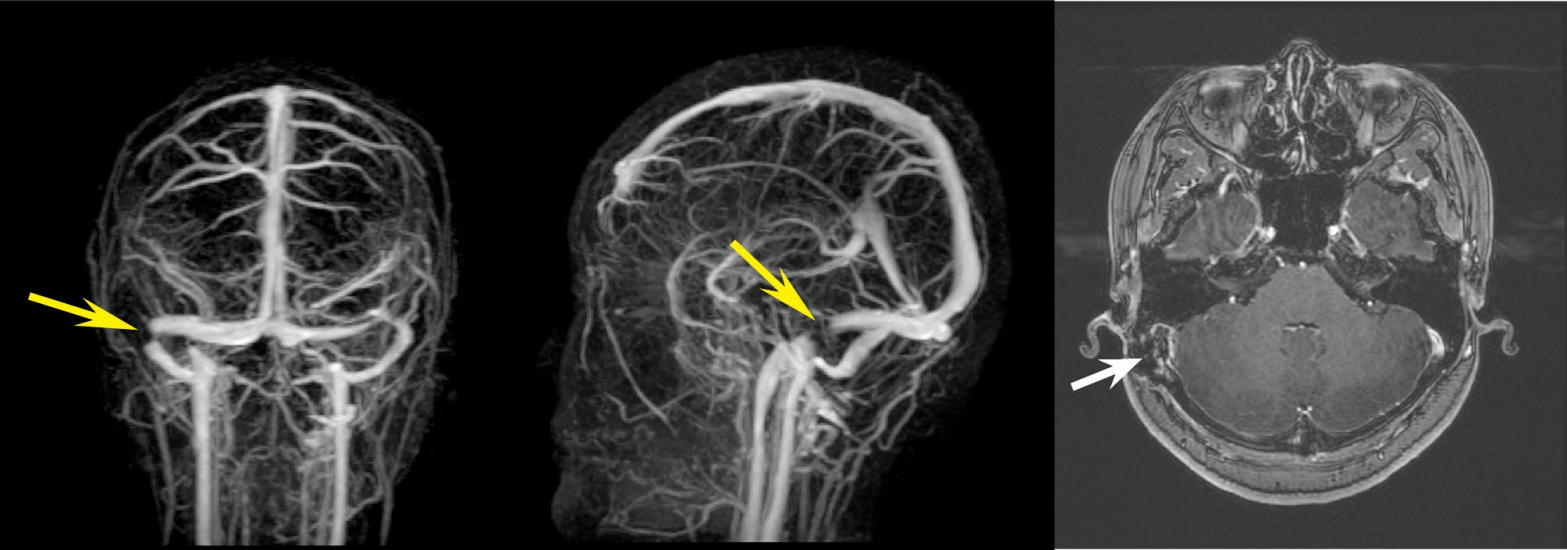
Magnetic resonance venography (MRV) showing the right sigmoid sinus filling defect shadow (yellow arrows), with a size of 13 mm*10mm. The same findings (white arrow) could be seen in contrast-enhanced magnetic resonance imaging (MRI)
